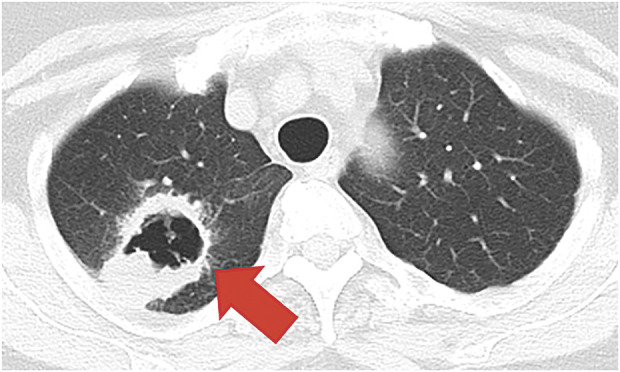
Chest computed tomography before treatment with chemotherapy and immune checkpoint inhibitor. A 31-mm mass with a cavity (red arrow) was detected in the right upper lobe of the lung. The pathological diagnosis was squamous cell lung cancer.
